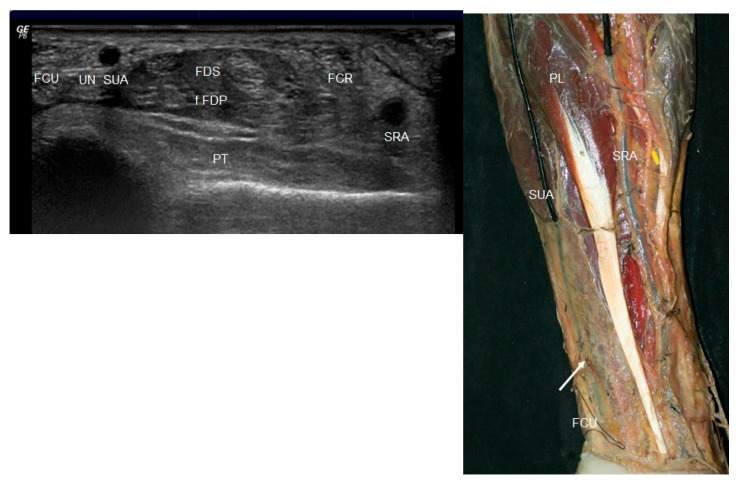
(Left) Distal ultrasound view of the forearm with the superficial ulnar artery (SUA) and the superficial radial artery (SRA) in relation to the flexor carpi ulnaris (FCU) and ulnar nerve (UN) flexor carpi radialis (FCR) and pronator quadratus muscle (PT). Flexor digitorum superficialis (FDS) and flexor digitorum profundus (FDP). (Right) Dissection of the anterior side of the forearm it could see the palmaris longus (PL), the flexor carpi ulnaris (FCU) and the superficial ulnar artery (SUA) and the superficial radial artery (SAR) under the forearm fascia was not dissected in the inferior third of the forearm.
